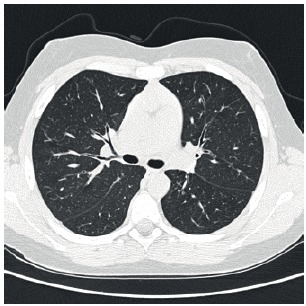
HRCT scan of the chest showing pronounced ground-glass changes in both lung
